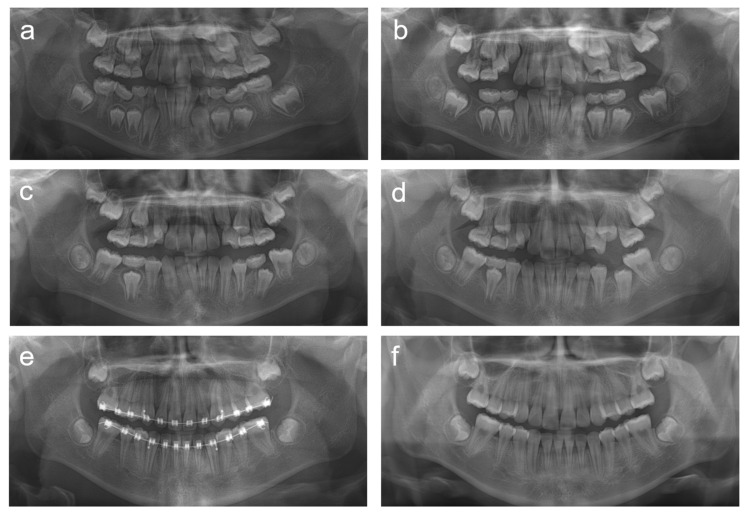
Panoramic radiographs of patient 5. (a) Panoramic radiograph at age 8 years 10 months. (b) Panoramic radiograph at age 9 years 8 months. (c) Panoramic radiograph at age 10 years 9 months. (d) Panoramic radiograph at age 11 years 2 months. (e) Panoramic radiograph at age 12 years 11 months. (f) Panoramic radiograph at age 14 years 1 month.
